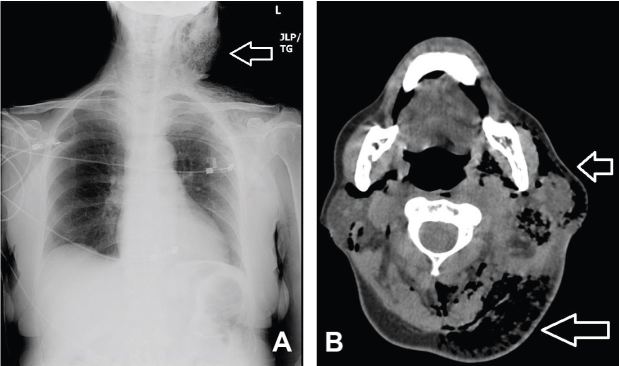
A*, Chest radiograph and *B*, neck computed tomography image at level of C2, both demonstrating left-sided neck mass with extensive subcutaneous emphysema (open arrows).
